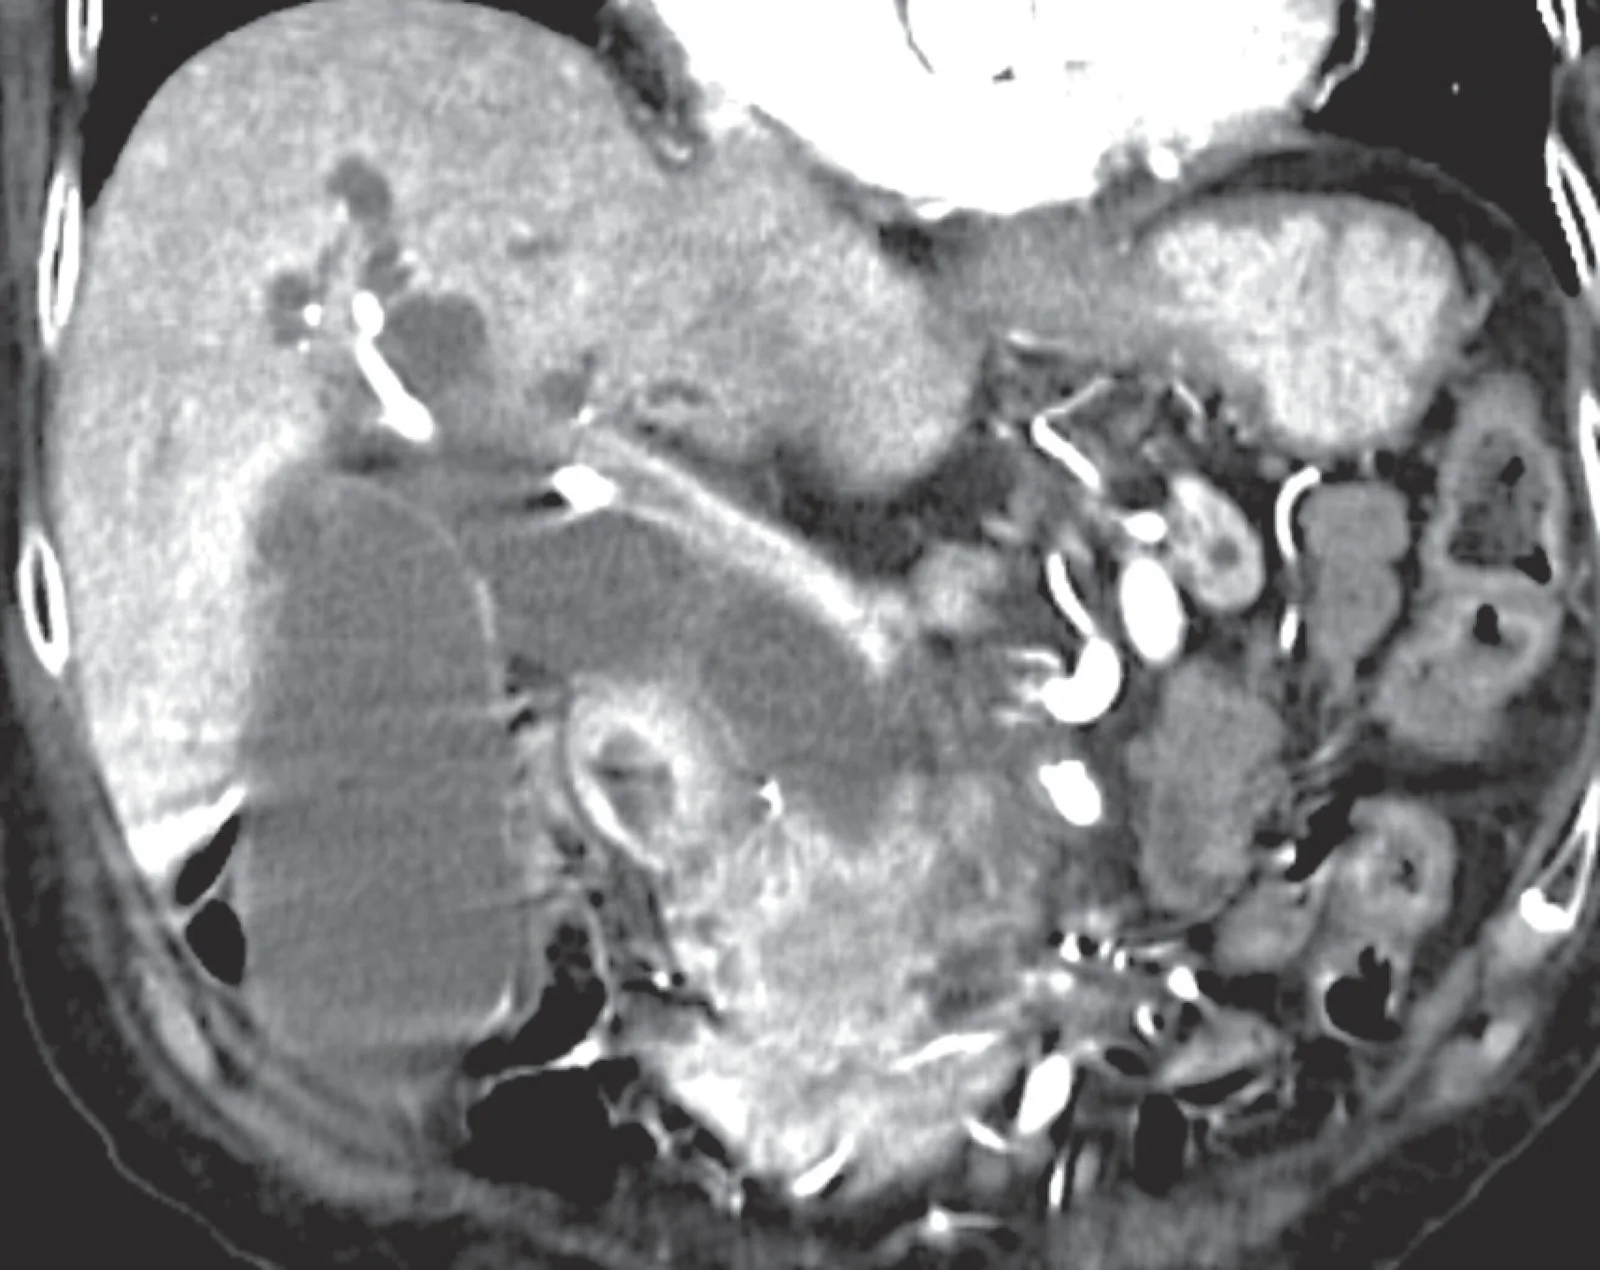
Contrast-enhanced computed tomography shows distal biliary
